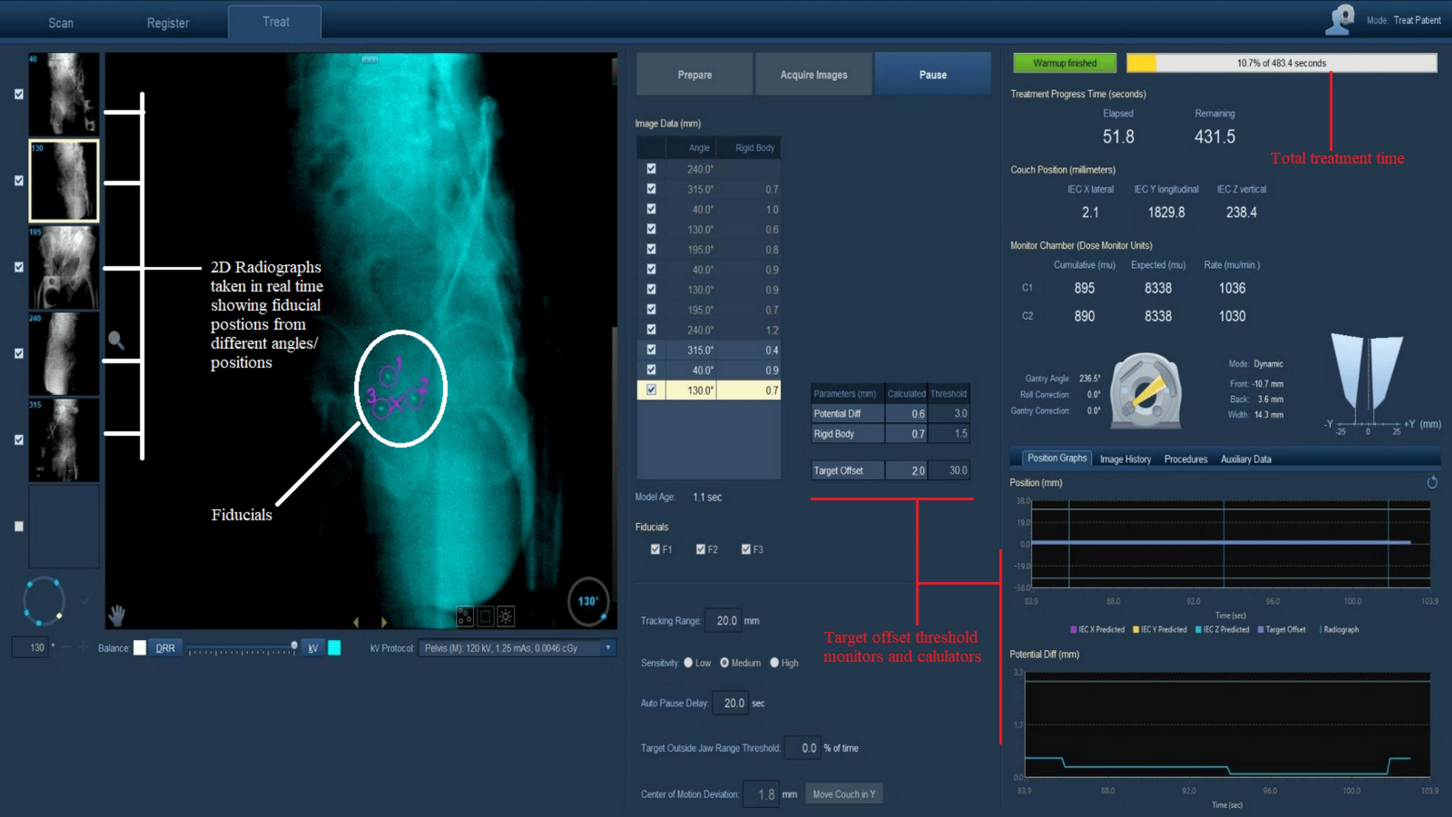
Intra-fraction motion management using Synchrony® for tracking the fiducial position using 2D radiographs from multiple positions continuously in real time
